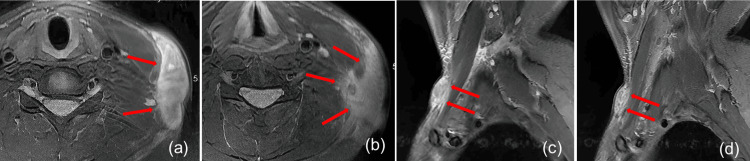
MRI findings on April 8, 2023 On the surface of the left neck, an irregular mass is visible, mainly involving the subcutaneous tissue but with an unclear boundary with the deep fascia, and some areas have invaded the deep fascia. In cross-section, the size of the mass is about 8.9 x 2.1 cm, with an up-down diameter of approximately 2.0 cm. It shows a high signal on T2WI. The MRI examination reported that the mass in the left neck may be nodular fasciitis. (a, b, cross-section; c, d, sagittal section) MRI: magnetic resonance imaging
